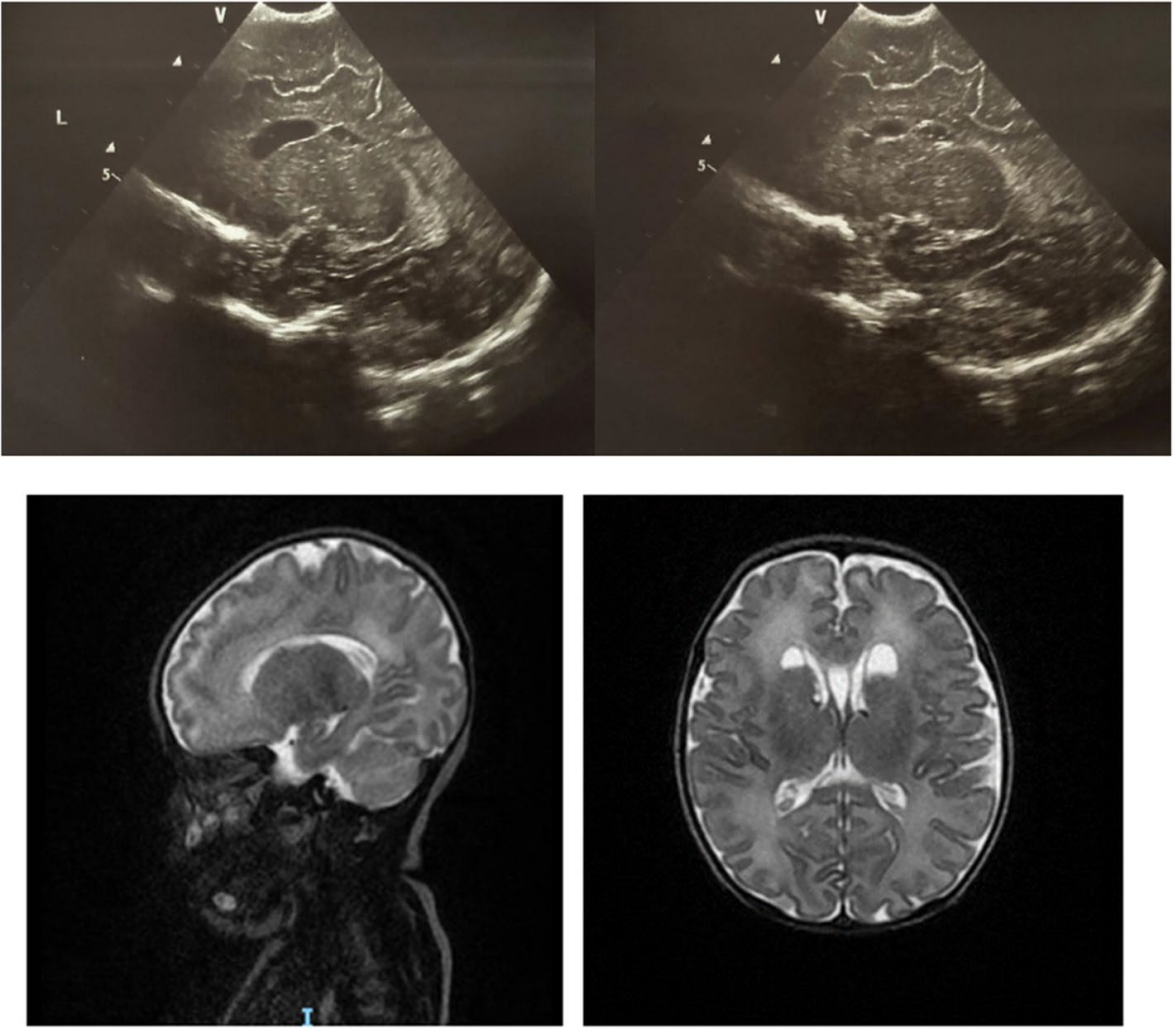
cUS and T_2_-weighted sequences of the brain MRI of a term female newborn exposed to SARS-CoV-2 in utero at 22 W showing bilateral FHCs and bilateral subependymal cysts
